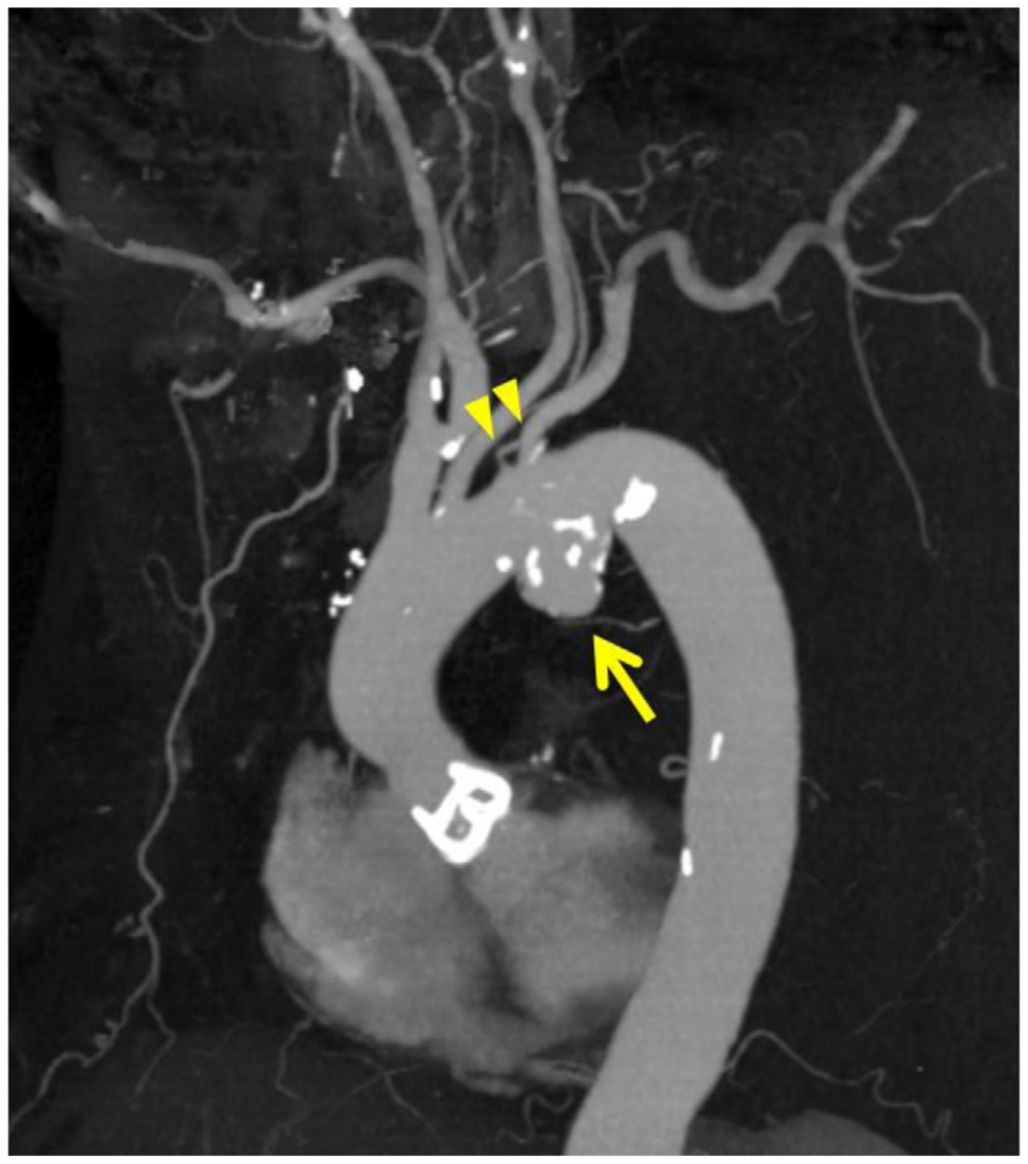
Three-dimensional reconstruction of the thoracic aorta from computed tomography (CT) angiography before thoracic endovascular aortic repair (TEVAR). CT angiography showed a saccular aneurysm of the aortic arch (*arrow*). The maximum diameter of the aneurysm was 52 mm. The left vertebral artery branched directly from the aorta. The left subclavian artery and left vertebral artery (*arrowheads*) branched at the same level as the aortic aneurysm.
